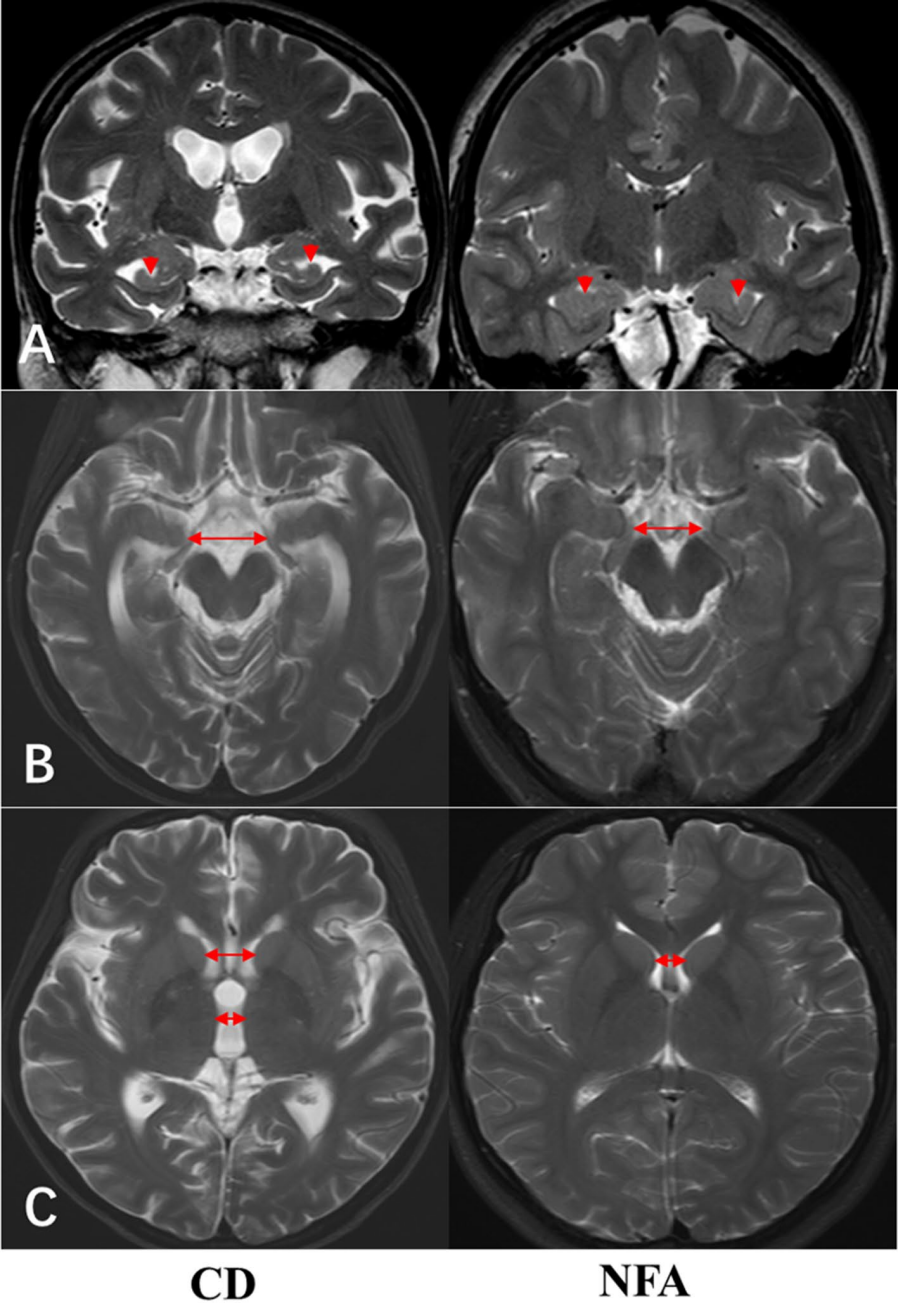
(A) MRI Image of a 43-year-old female CD patient with hippocampal atrophy compared with NFA subject; (B) MRI Image of a 45-year-old female CD patient with temporal lobe atrophy compared with NFA subject; (C) MRI Image of a 40-year-old female CD patient with subcortical atrophy compared with NFA subject.
